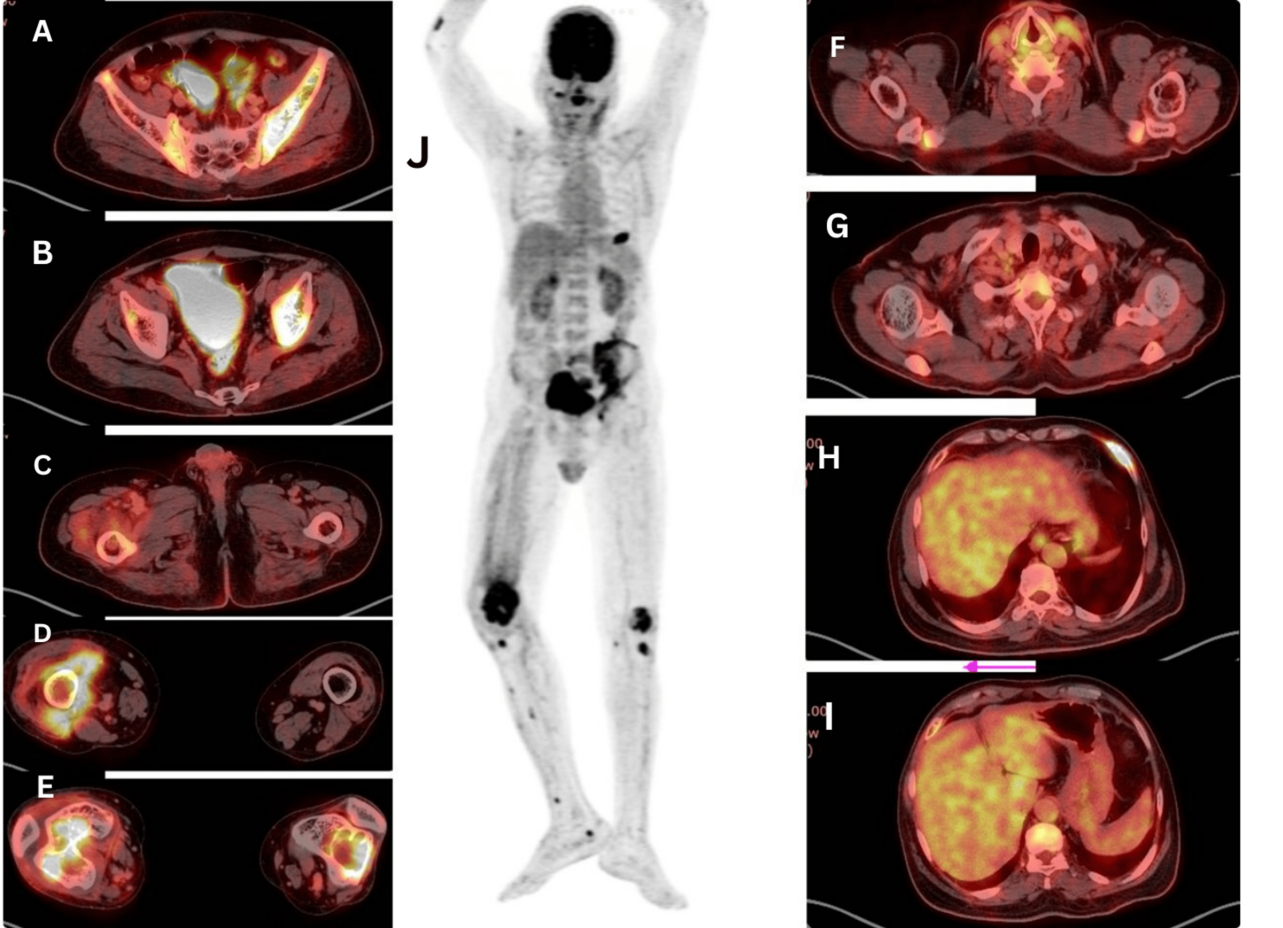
(A–C): Axial PET-CT images of the pelvis reveal a metabolically active lesion involving the left iliac bone, iliacus muscle, sacroiliac joint, and extending to the left acetabulum. (D, E): Axial images of the thigh show an extensive osteolytic lesion in the right femoral condyle extending into the articulating surface with associated soft tissue involvement, along with a similar lesion in the left femoral condyle. Axial images of the neck (F, G) and liver (H, I) regions show no metabolically active focal lesion. (J): 18F-FDG PET-CT highlights metabolically active osteolytic lesions in the left anterior sixth rib, left iliac bone, sacroiliac joint, and bilateral femoral condyles with adjacent soft tissue involvement on the right. Focal metabolic activity is also noted in the proximal bilateral tibial condyles.
